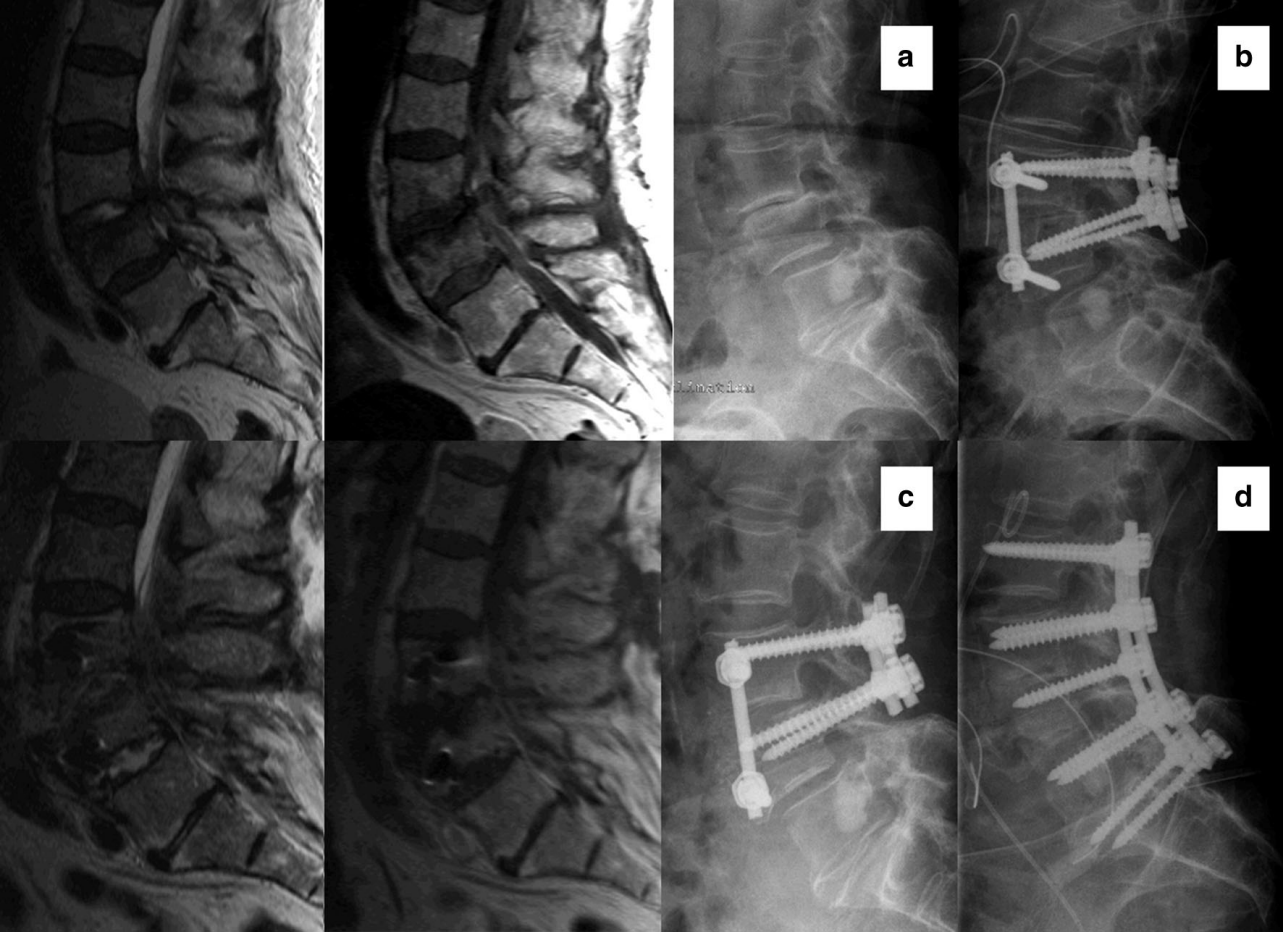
Patient 12: sagittal MRI cuts T2- and T1-weighted, and lateral radiographs; a preoperatively, b after primary operation, c adjacent segment infection, and d after ASI surgery
